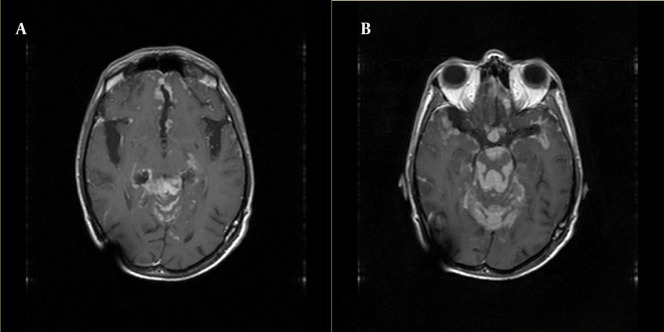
A and B, Axial brain contrast enhanced T1-weighted magnetic resonance images demonstrate nodular and diffuse enhancing regions of leptomeninges throughout basal cisterns and sulci in the interhemispheric fissure, in the location of presumed melanocytoma lesions.
